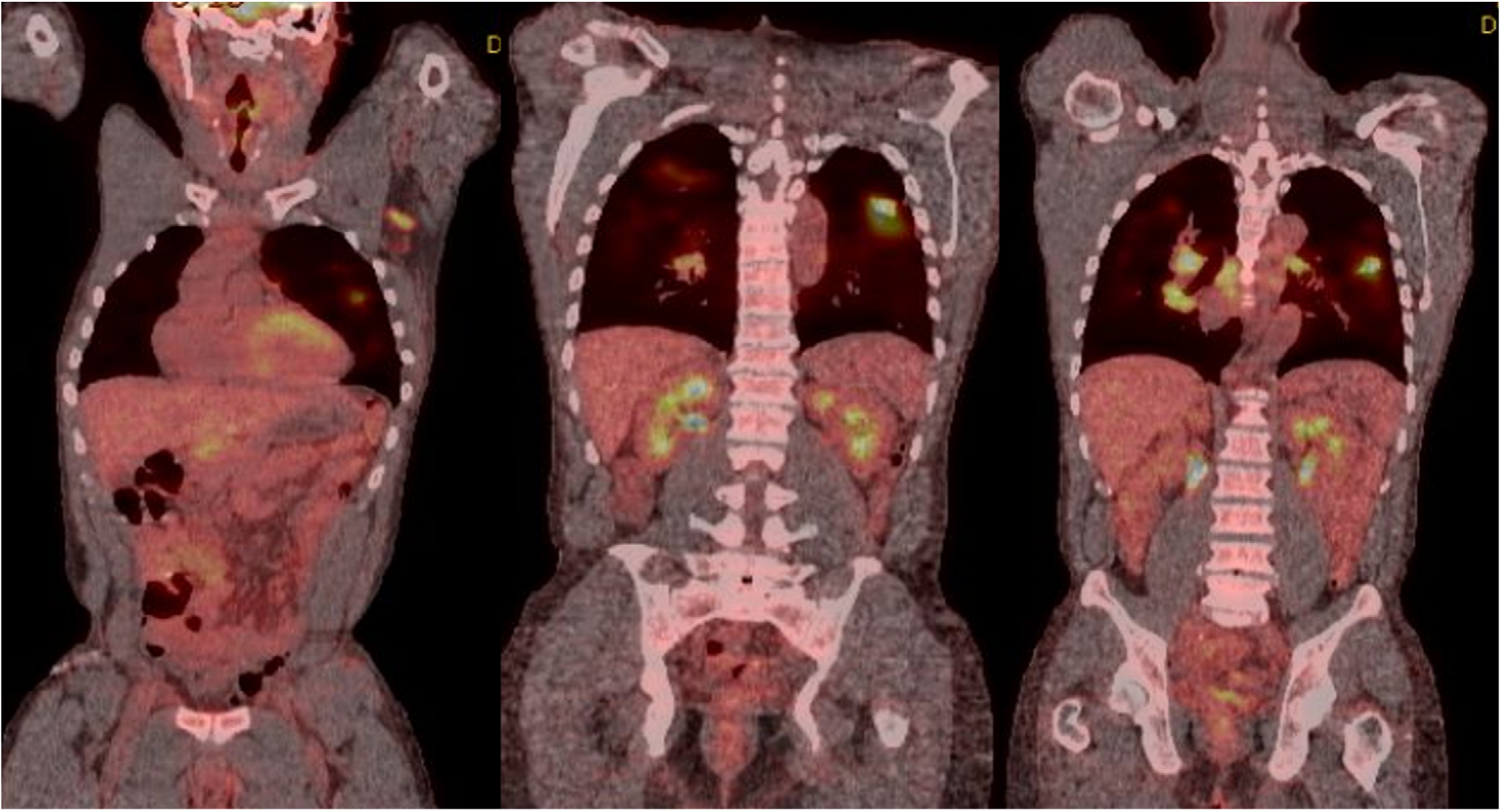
F‐18 fluorodeoxyglucose (FDG) positron emission tomography (PET) demonstrated metabolically active supraclavicular, axillary, mediastinum, and hilar lymph nodes. There was also hypermetabolic activity in the left lung nodule and mesenteric mass in the abdomen.
